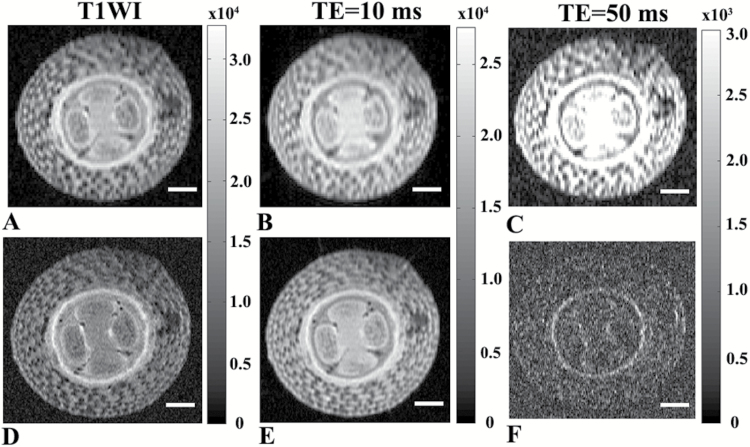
Relaxation-based MR images of tomato hypocotyls. Conventional MR images of 5-week-old tomato hypocotyls obtained with in-plane spatial resolutions of (A–C) 35 × 70 µm^2^ and (D-F) 17 × 35 µm^2^. (A, D) T1-weighed images collected with TR/TE of 700/15. (B, E) Short TE T2 MR images (TR/TE=3000/10), (C, F) Long TE T2-weighted images collected with TR/TE of 3000/50. Scale bars are 500 μm.
